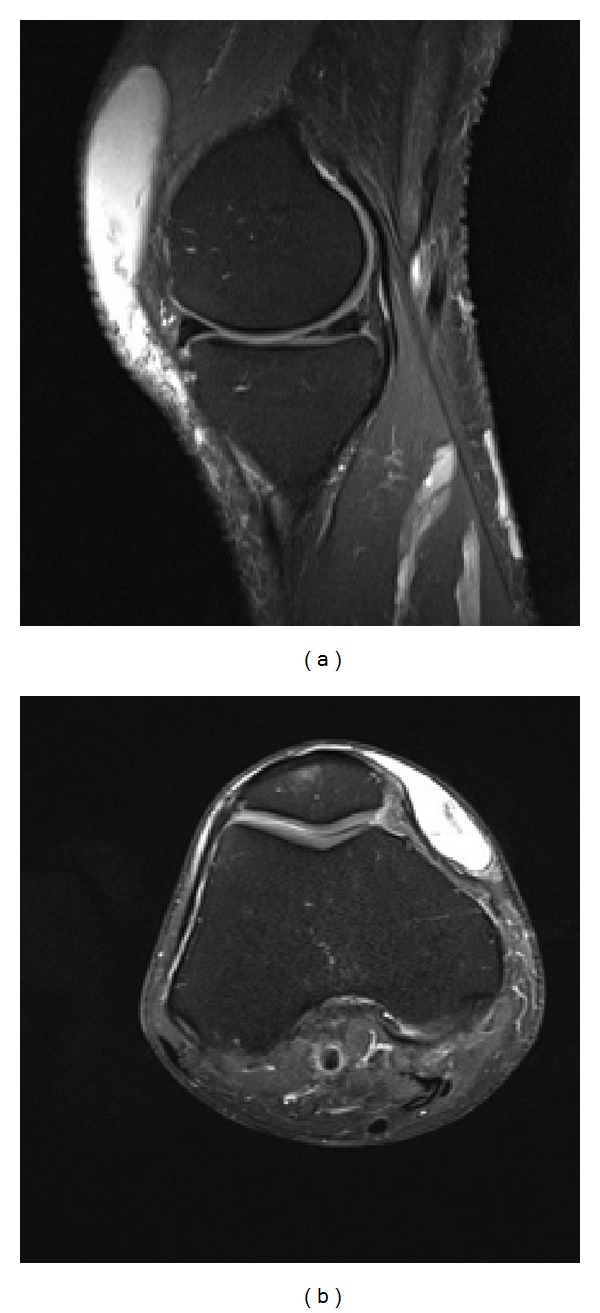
Magnetic resonance images (T2-weighted) highlighting the prepatellar collection of fluid in sagittal (a) and axial (b) sections.
